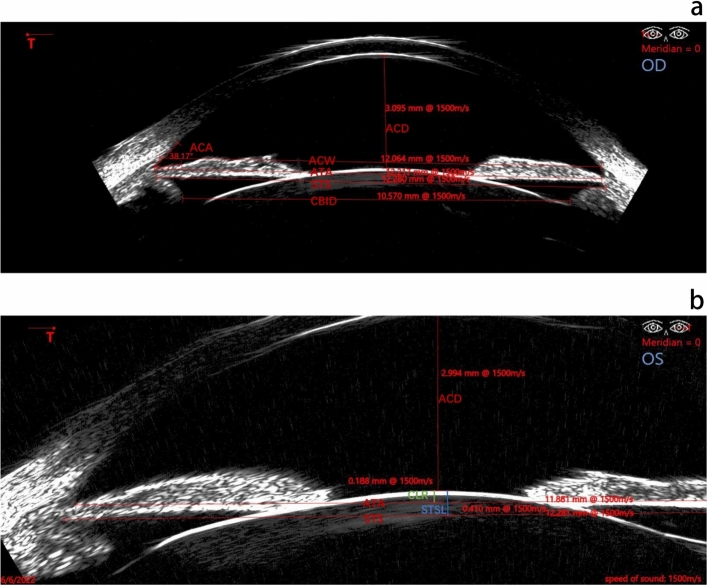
The parameters measured by Artemis Insight 100 very high-frequency digital ultrasound B scan. (a) Anterior chamber depth (ACD), anterior chamber angle (ACA), anterior chamber width (ACW), angle-to-angle (ATA) diameter, sulcus-to-sulcus (STS) diameter, ciliary body inner diameter (CBID); (b) the lens rise from the ATA plane described as crystal lens rise (CLR, shown as green) and the lens rise from the STS plane named STS lens rise (STSL, show as blue).
